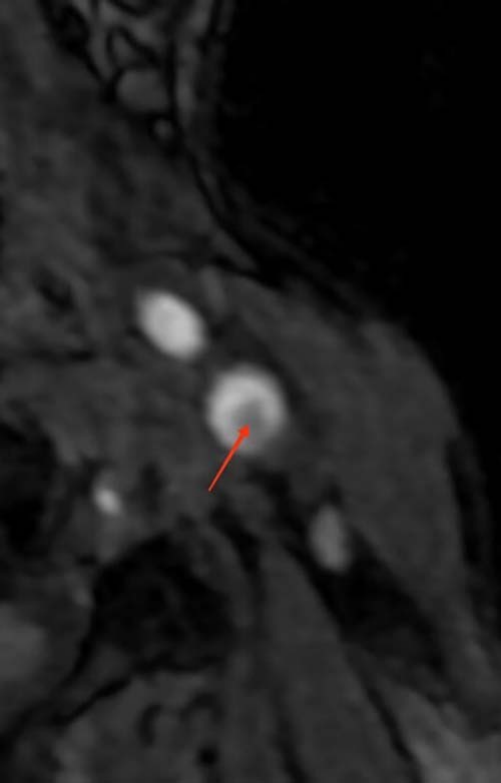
Cross-section showing a bulbar thrombus (MRI).
